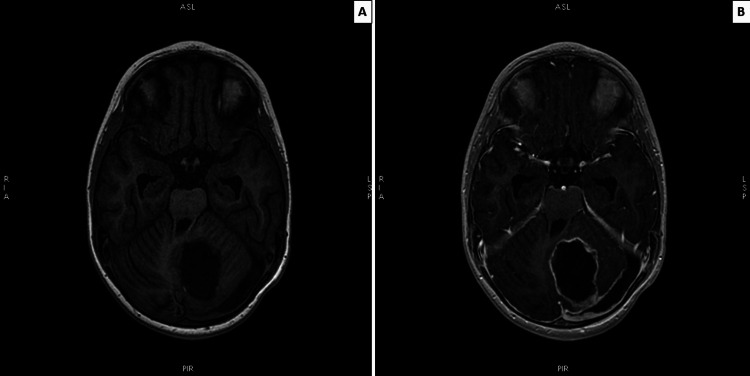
(A) AX 3DT1 native and (B) AX 3DT1 postcontrast MRI of the brain showing postoperative changes in the left cerebellar hemisphere-porencephalyc cyst with peripheral gliosis and hemosiderin deposits, with peripheral contrast enhancement AX 3DT1: axial three-dimensional longitudinal relaxation time; MRI: magnetic resonance imaging
